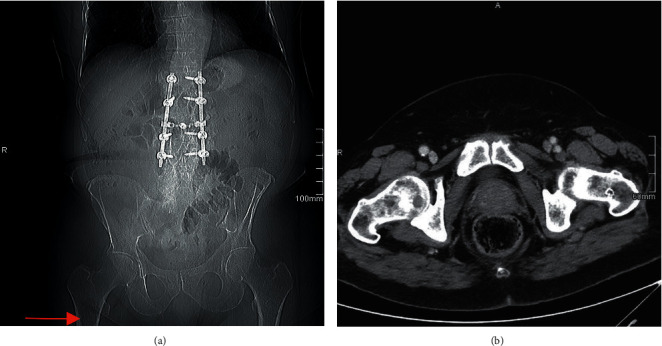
Imaging of a patient with an unobserved pathologic lesion who went on to complete fracture. (a) Routine surveillance scout CT film demonstrating a pathologic lesion with an impending fracture in the right subtrochanteric femur that was not documented in the initial radiographic report. (b) An axial CT view in which the lesion identified by authors in the scout images is not well visualized and was not described in the formal radiographic report. These are of a 76-year-old patient with known metastatic renal cell carcinoma being managed on pazopanib chemotherapy per oncologic specialists.
